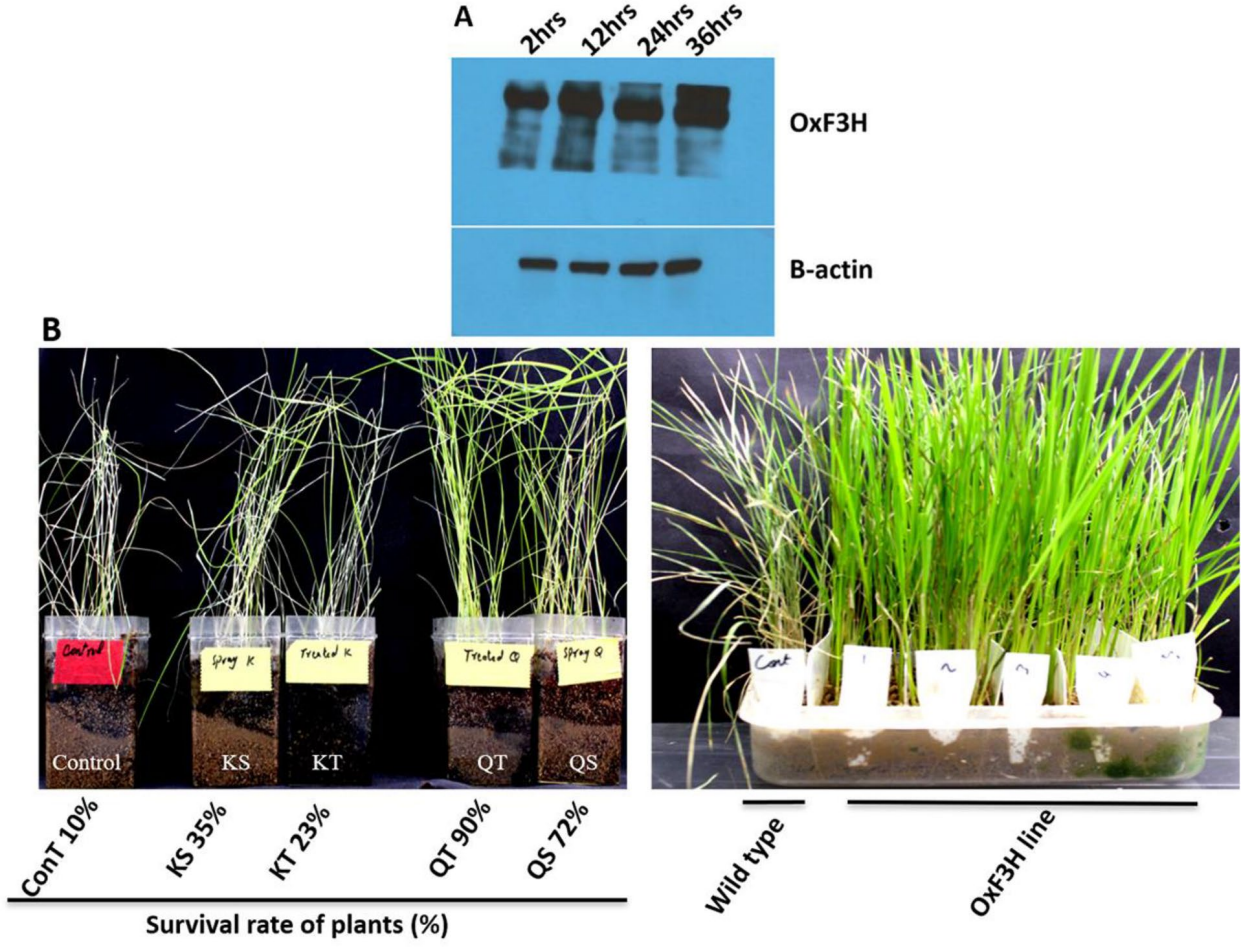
Effect of exogenous application of kaempferol and quercetin on survival rate of plants using susceptible line TN1 and survival difference between wild type and transgenic non-treated plants along with immunoblot analysis of *OsF3H* protein accumulation in transgenic plants. (A-upper) Expression of *OsF3H* protein during 2, 12, 24 and 36 h of WBPH infestation to transgenic plants analyzed by western blotting using gene specific antibody. (A-lower) β-actin of infected transgenic plants. The β-actin was run with the same sample on the same blot corresponding to the *OsF3H* blot. Figure (A) was more cropped because same sample was run in two replicates in separate gels with other samples due to limited time and resources and then transferred to one X-ray film and again replicated on another X-ray film to make it more clear (Figure S8). The blots were cut after the protein transfer to incubate with different antibodies to save time, reagents and materials. (B-left picture) KS is kaempferol sprayed, KT is kaempferol treated, QT is quercetin treated and QS is quercetin sprayed. Both the compounds were applied in 3 ppm quantity. The best result shown by quercetin treated and sprayed however, the result of kaempferol treated and sprayed was also better than control plants. (B-right picture) Represent the effect of WBPH on susceptible and transgenic line. Control was TNI susceptible to WBPH and the rest represent *OxF3H* line 1–5.
